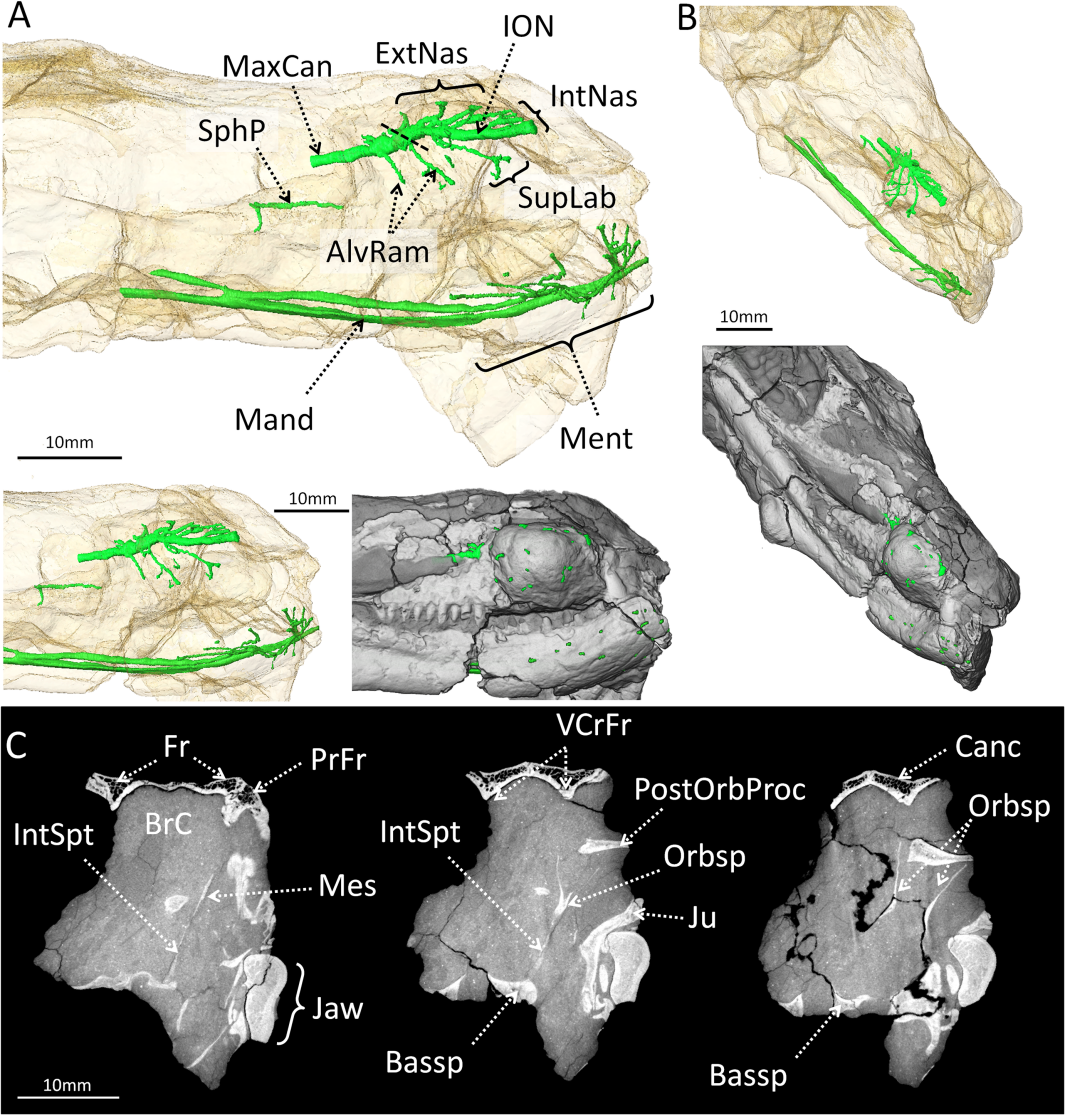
Three-dimensional reconstruction of the maxillary canal, mandibular canal, sphenopalatine canal and brain-case of *Choerosaurus dejageri*. A, Lateral view with the skull transparent (top) and non-transparent (below). B, oblique view with the skull transparent (top) and non-transparent (below). The trigeminal nerve occupies the majority of these canals [52, 53], and the identification of trigeminal branches is based on the name of the corresponding nerve in mammals. C, CT images of the transverse section throught the braincase of *Choerosaurus dejageri* (from left to right: rostral to caudal sections). Abbreviations: AlvRam, alveolar rami; Bassp, basiosphenoid; BrC, braincase; Canc, cancellous bone; ExtNas, external nasal rami of the infraorbital nerve; Fr, frontal; IntNas, internal nasal rami of the infraorbital nerve; IntSpt: interorbital septum; ION, infraorbital nerve; Jaw, lower jaw bones; Ju, jugal; Mand, mandibular rami; Mes, mesethmoid; Ment, mental foramina; MxCan, maxillary canal; Orbsp, loose orbitosphenoid; PrFr, prefrontal; PostOrbProc, loose postorbital process; SphP, sphenopalatine rami; SupLab, supralabial ramus of the infraorbital nerve; VCrFr, ventral crests of the frontal. Scale bar: 10 mm.
